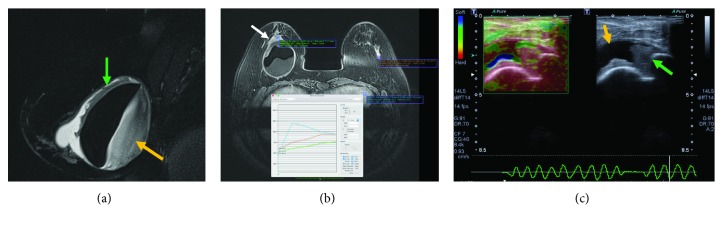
Mixed SIGBIC with seroma: imaging findings. (a) BMRI. Sagittal T2-weighted sequence presenting a large seroma with thick seroma content (yellow arrow). There are also enhanced masses on the anterior surface (green arrow). (b) Dynamic postcontrast sequence. Extracapsular mass (white arrow) that presents the type I kinetic curve pattern (persistent—green curve), inferring vascularized lesion. (c) Ultrasonography with elastography. Orange arrow points to the seroma component and the green arrow to the solid component. The seroma component is soft by elastography while the solid is hard. The radiological differential diagnosis are infectious disease and BIA-ALCL.
